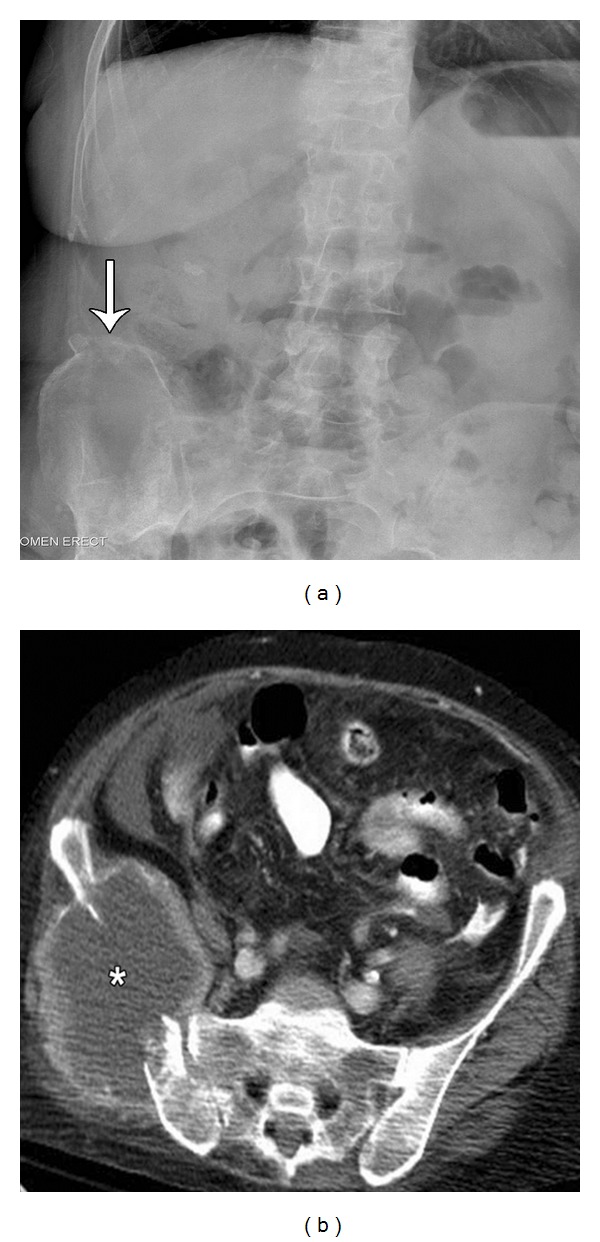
Middle-aged female presents with chronic pelvic pain. Frontal radiograph (a) of the abdomen reveals subtle cortical disruption and periosteal reaction involving the superior aspect of the right iliac crest (arrow). Subsequent CT examination (b) demonstrates a lytic destructive lesion of the right iliac bone, with a large soft tissue mass (asterisk).
